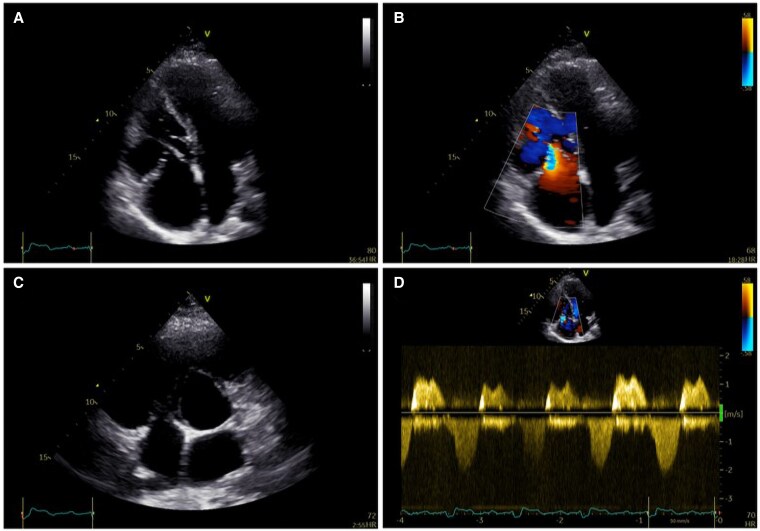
(*A*, *B*) Apical four-chamber view showing a well-functioning tricuspid valve after repair by 2D transthoracic echocardiography (*A*) and mild to moderate tricuspid regurgitation with Doppler imaging (*B*). (*C*) Short axis view with a focus on tricuspid valve showing good motion of tricuspid valve leaflets and the presence of the spontaneously closed VSD. (*D*) Continuous wave Doppler by transthoracic echocardiography at the tricuspid valve after repair depicting transvalvular mean gradient of ∼3 mmHg at 70 b.p.m. in the presence of mild to moderate regurgitation. RV, right ventricle; VSD, ventricular septal defect.
